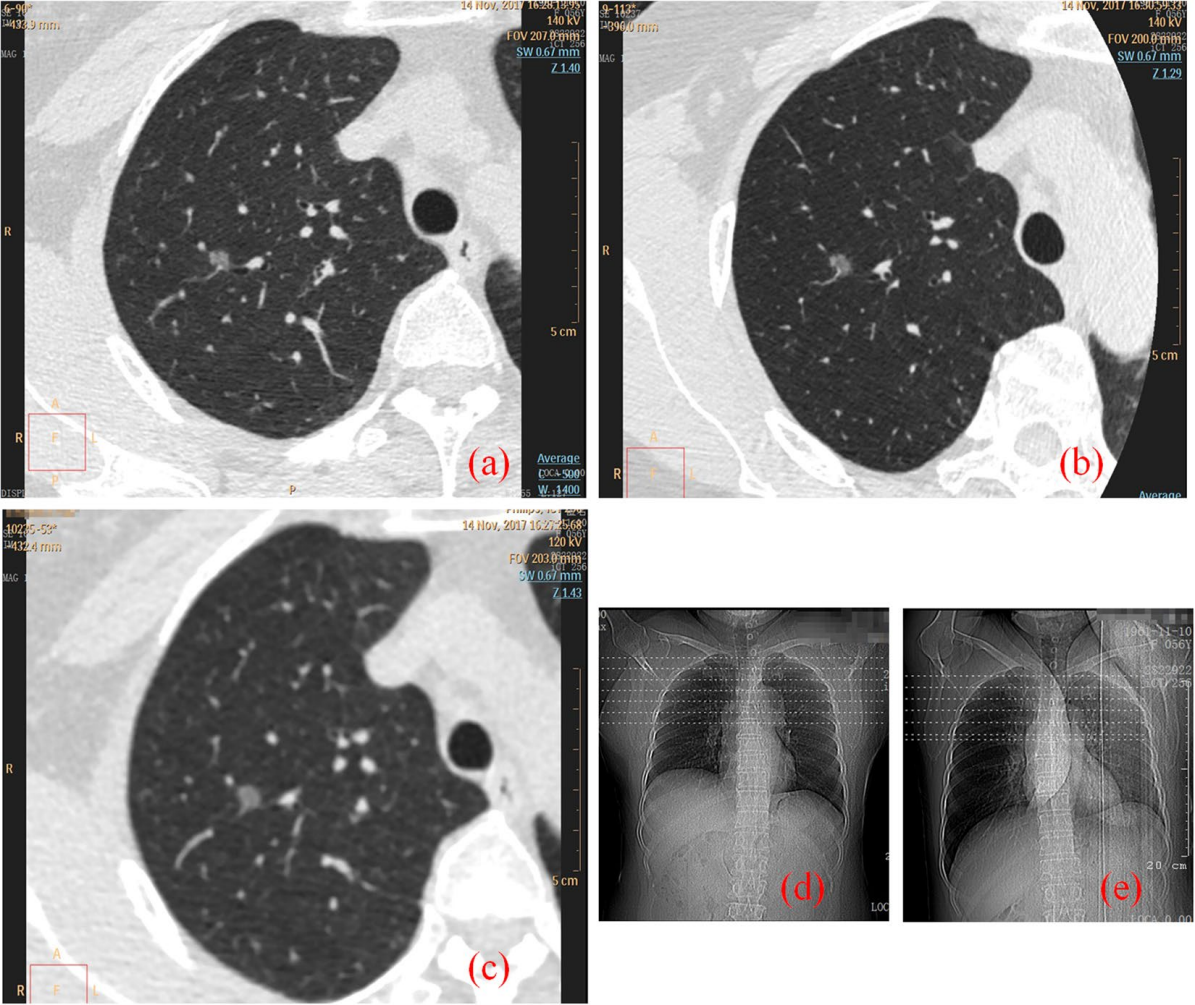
Comparison of nodule cross-sectional imaging for the three CT scanning schemes. The nodule was located in the lung. The images were provided by department of radiology of Jinhua Central Hospital to Zhejiang University and were from a 57-year-old female patient. (a,d) 1024 ultra-high-resolution scan with patients stay in supine position (B-Scan). (b,e) Breath-hold assisted ultra-high-resolution scan with patients lie in lateral position (G-Scan). (c,d) Retrospective 1024 matrix target scan with patients stay in supine position (A-Scan).
